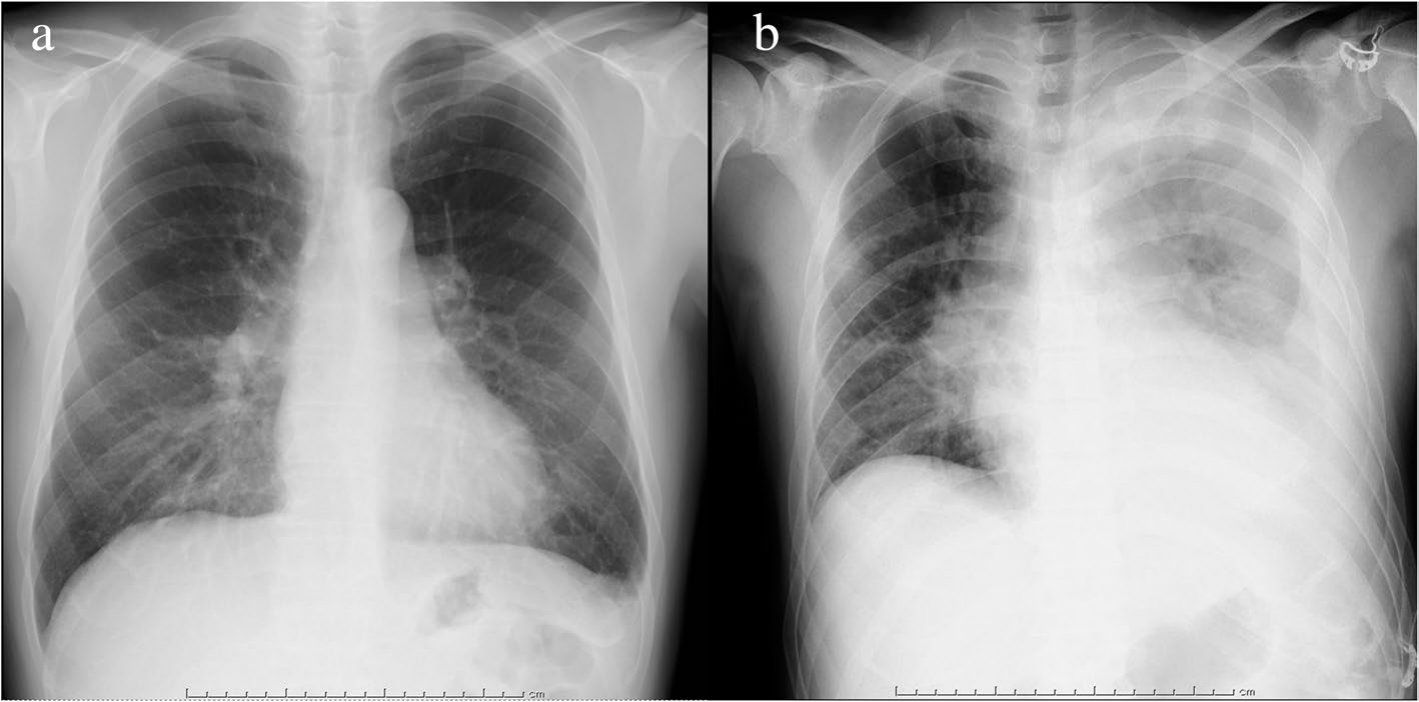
a Chest radiograph image taken 27 days before surgery, showing a small left pleural effusion. b Chest radiograph image taken immediately following surgery, showing a large volume of left pleural effusion
